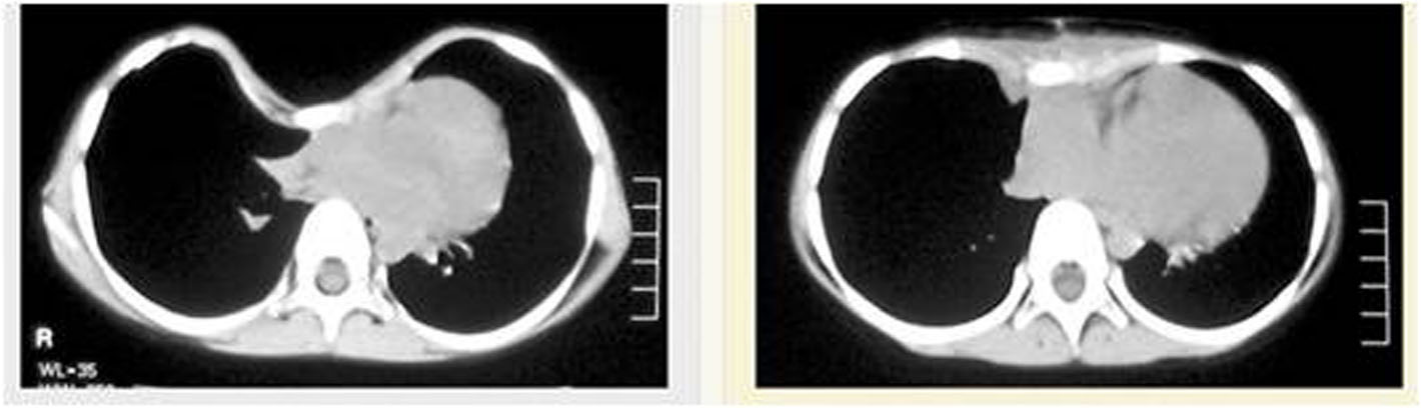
Plain CT chest pre-operative and post-operative follow up
